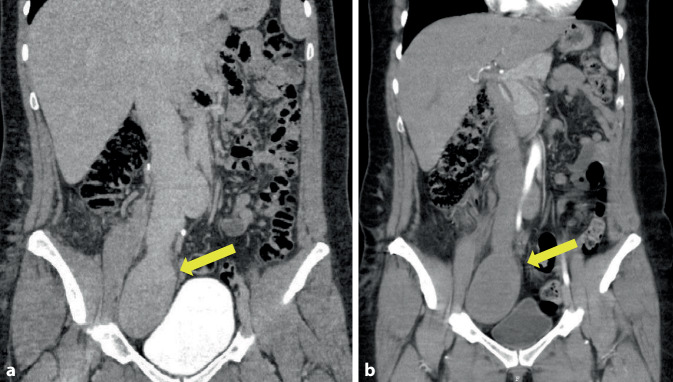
Fall 7: Aneurysma der rechten Beckenvene und V. cava inferior. a CT-Angiographie in koronarer Schichtung bei Erstdiagnose des venösen Aneurysmas der V. iliaca und V. cava inferior (*gelber Pfeil*), b CT-Angiographie-Verlaufskontrolle (koronar) nach 10 Jahren (*gelber Pfeil*)
